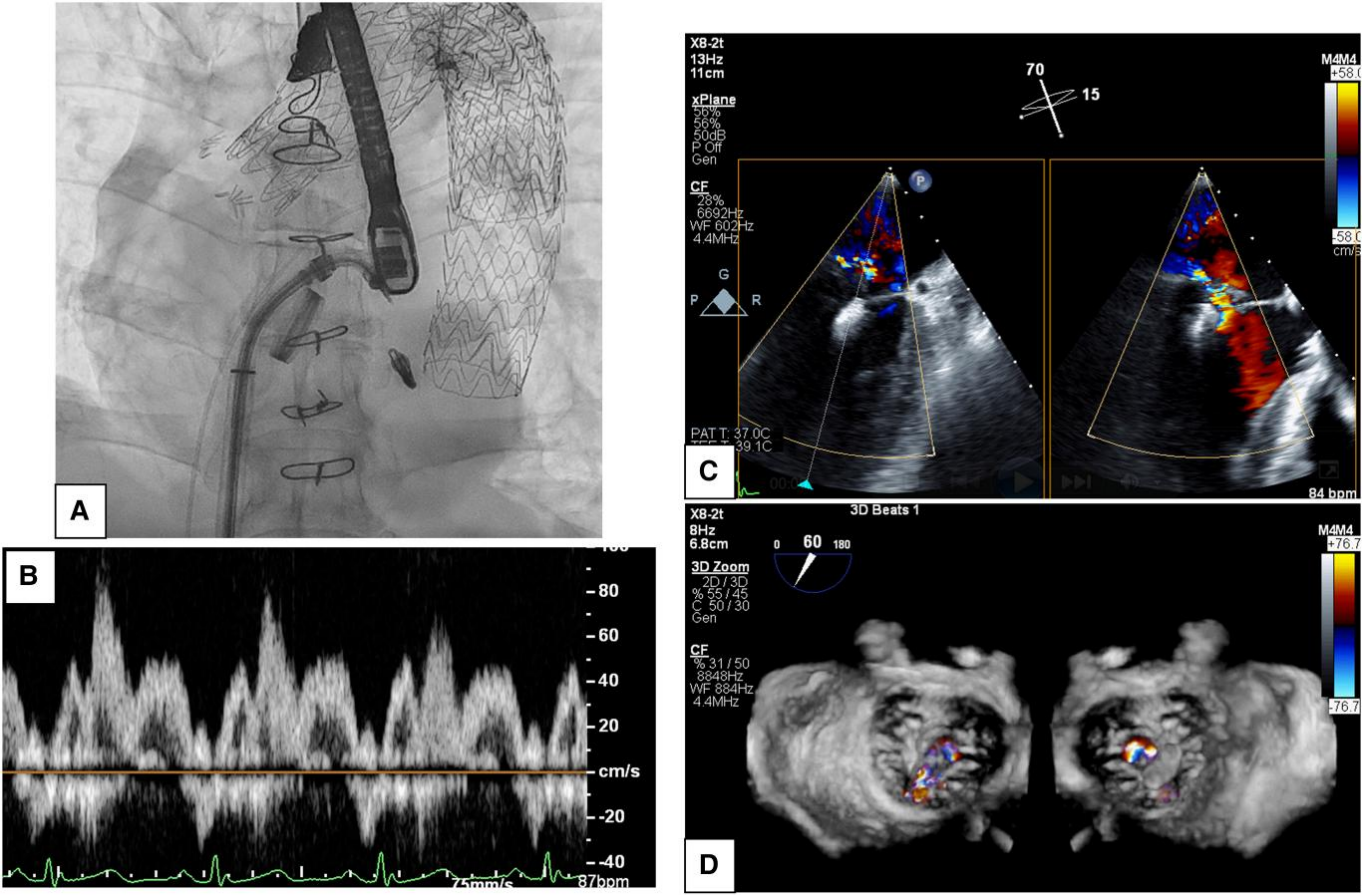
Intraprocedural findings of radiography and transoesophageal echocardiography. After the placement of the clip, mitral regurgitation is reduced but persists after single clip due to strain on the valve, resulting in a new indentation. (*A*) Fluoroscopic image after the placement of the clip. (*B*) Post-procedural pulse wave Doppler findings of the left superior pulmonary vein. Pulmonary vein systolic flow reversal is vanished and the inversion in the height of the S-wave and the D-wave is observed. (*C*) Mitral commissural view and left ventricle and aorta long axis view in TEE. Mitral regurgitation is reduced and remains mild-moderate regurgitation with placement of single clip. (*D*) Coloured three-dimensional of mitral valve view from left atrium and left ventricle on TEE. Clip is placed at the centre of AML2 and PML2 and a new indentation has occurred on the medial side of the clip, resulting in residual regurgitation from the same area.
